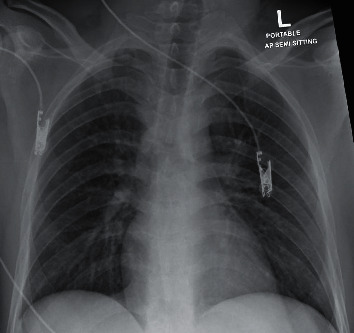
Chest X-ray on admission shows no lung infiltrates.
